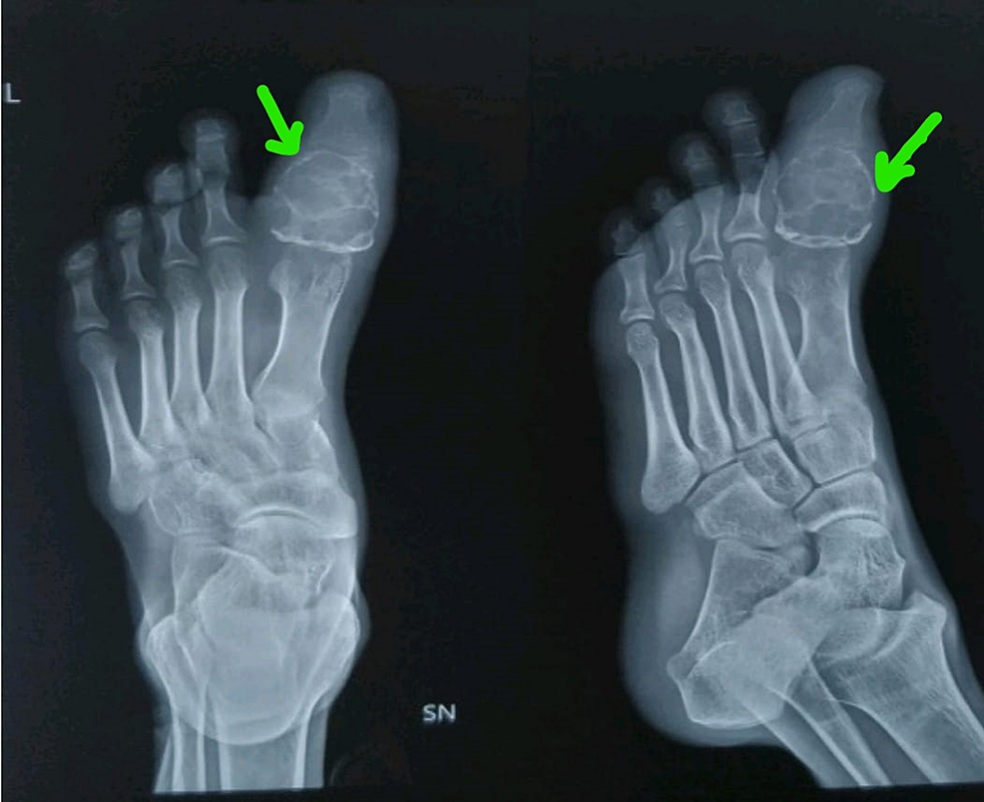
X-ray showing lytic lesions, scalloping of the cortex, and whorls of calcification.
